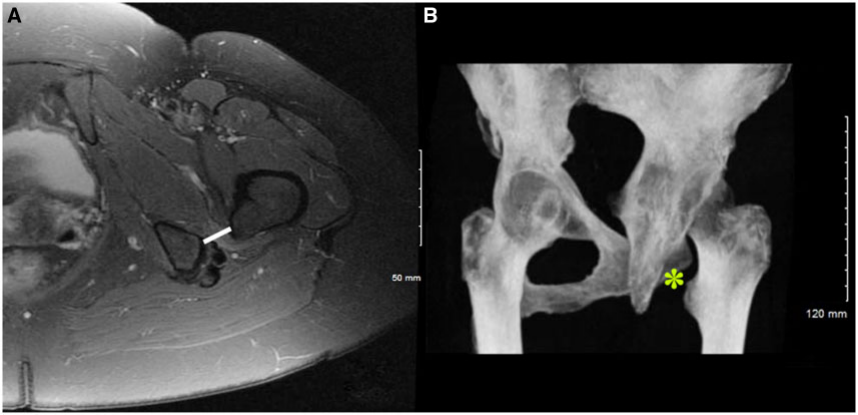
Radiographic assessment in a patient suspected with ischiofemoral impingement, demonstrating a narrow ischiofemoral distance on MRI (A) and 3D reconstructions (B) in combination with edema of the quadratus femoris muscle (A).
